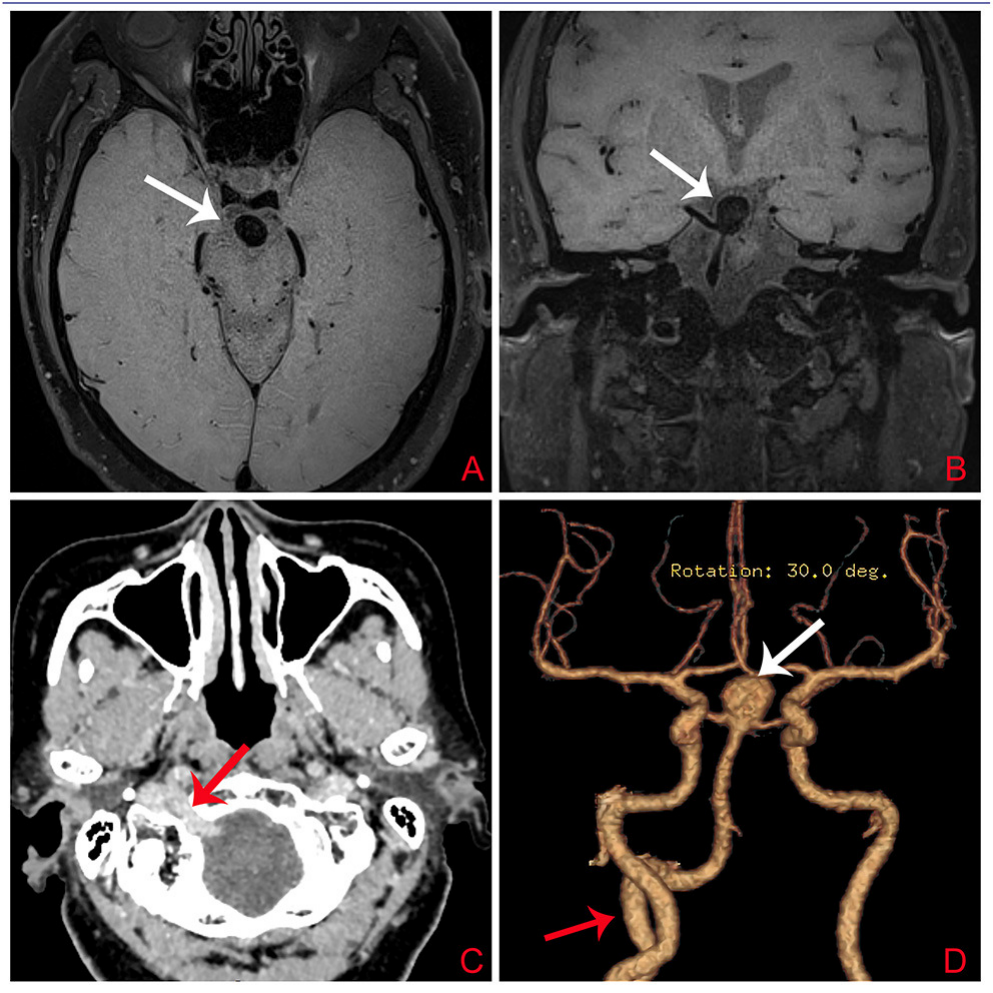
(A,B) T1-weighted image (T1WI) showing an aneurysm at the bifurcation of the basilar artery (white arrow). (C) Computed tomography angiography demonstrating the PPHA (red arrow) entering the skull after traversing the hypoglossal canal. (D) Computed tomography angiography showing the PPHA (red arrow) originating from the right ICA and joining the BA. An aneurysm (white arrow) located at the bifurcation of the basilar artery is visible. PPHA, persistent primitive hypoglossal artery; ICA, internal carotid artery; BA, basilar artery.
